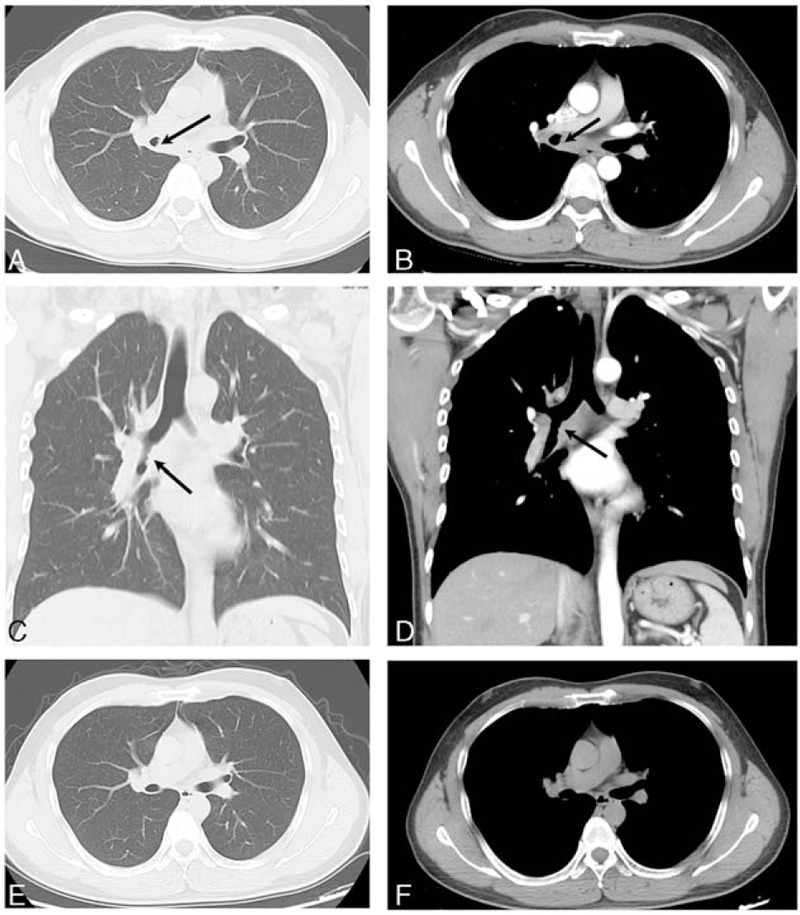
CT scan. (A) Enrollment into hospital; neoplasm in the bronchus intermedius (cross-section, lung window) (April 24, 2012). (B) Cross-section, mediastinal window. (C) Coronal section, lung window. (D) Coronal section, mediastinal window. (E) After 1.5 years; normal bronchus intermedius (cross-section, lung window) (November 5, 2013). (F) Cross-section, mediastinal window (November 5, 2013).
